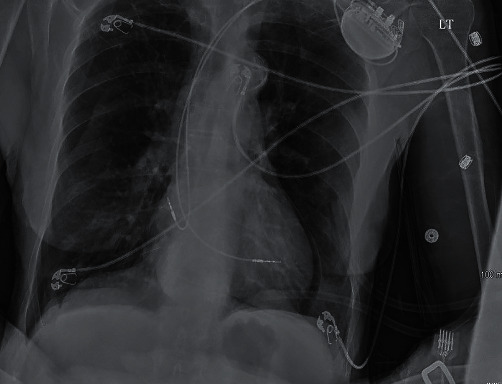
Anteroposterior chest radiograph revealing pneumopericardium.
